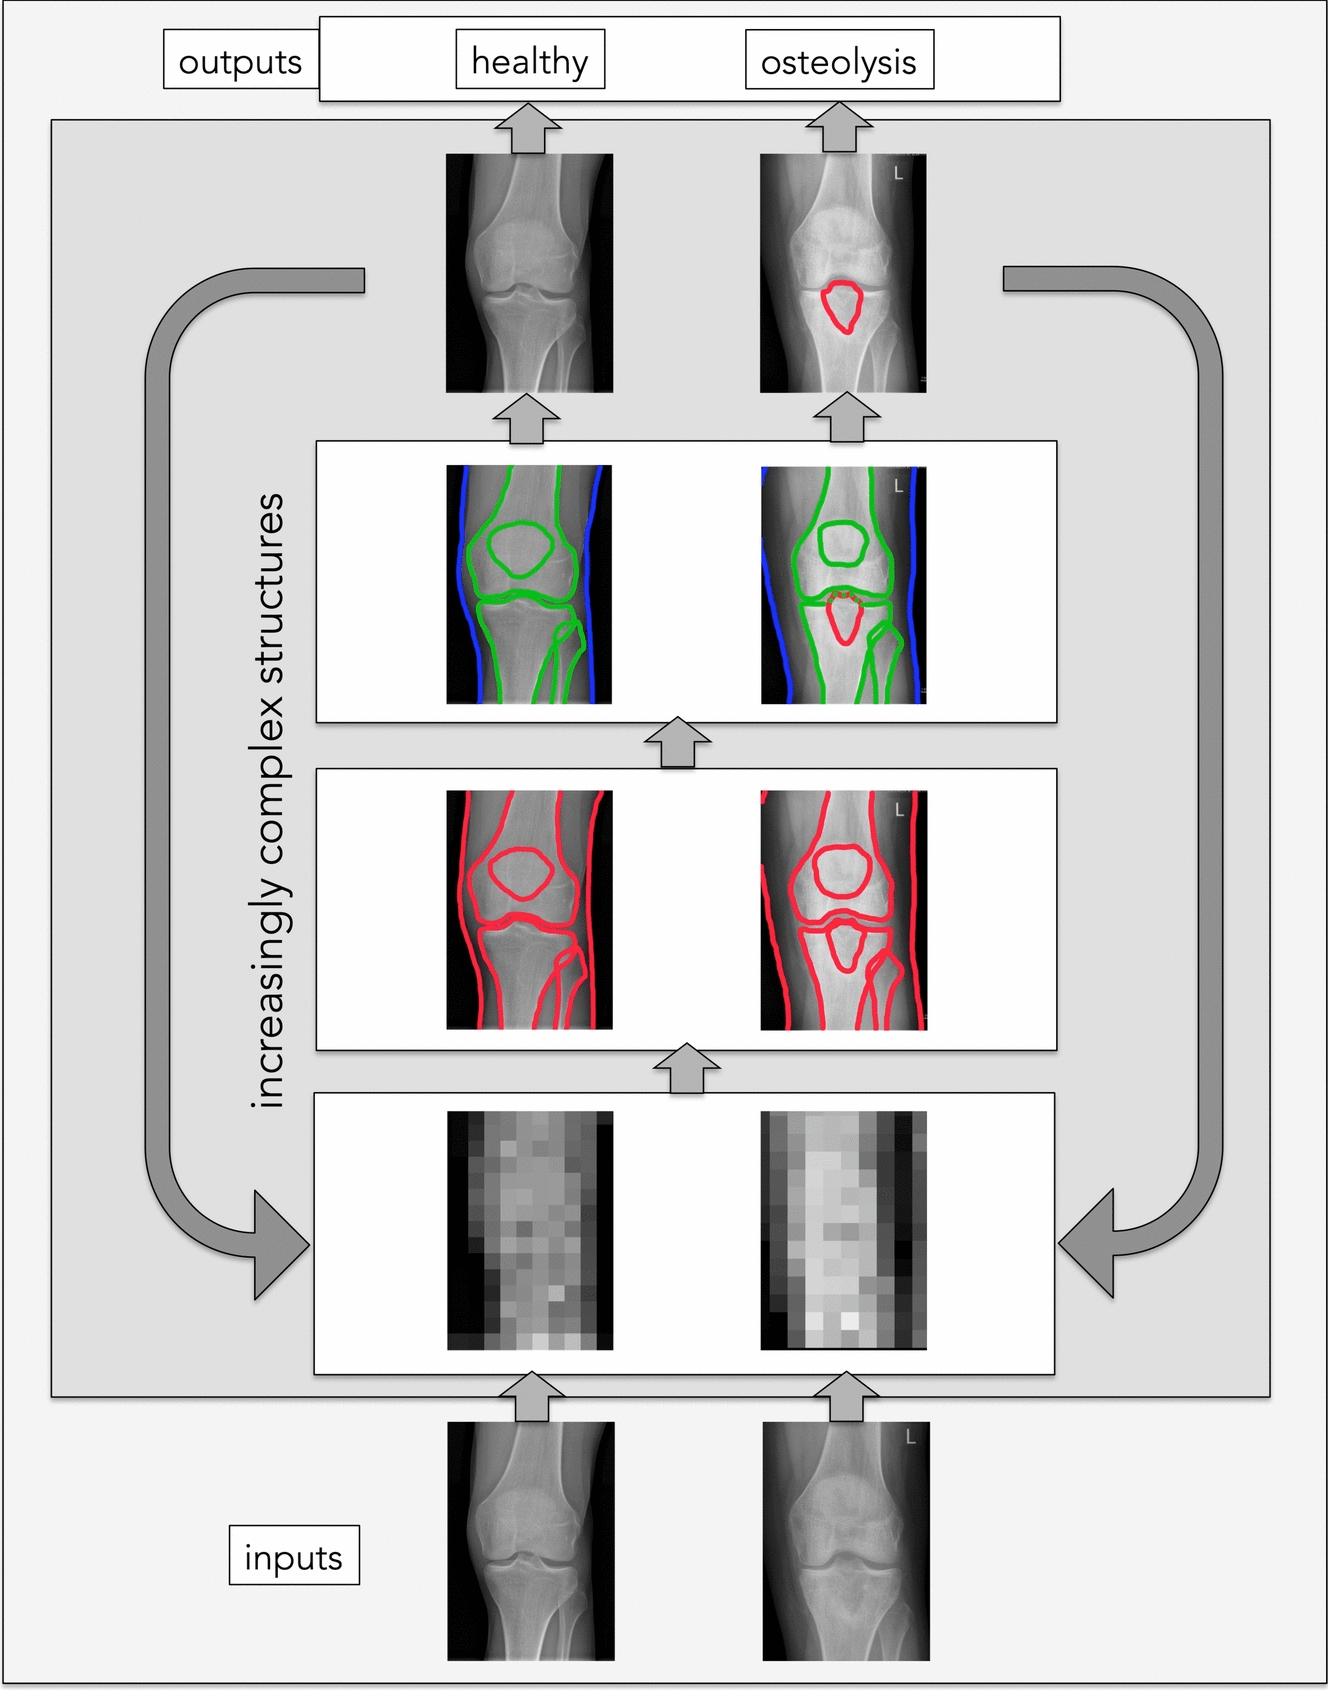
Machine learning model to detect osteolysis in a plain knee radiograph. Labelled input radiographs of healthy and pathological knees are given to the system. The training model then decomposes these images into grey value pixels. The model defines edges at areas of transition from higher to lower grey values. These edges are then aligned with the already-learned anatomy of a healthy knee radiograph. This feature extraction process involves identifying and capturing essential healthy and pathological knee characteristics. Aberrant lines are finally marked and labelled as pathologic. For the model creation, this process is repeatedly iterated to improve the diagnostic value of the model further
